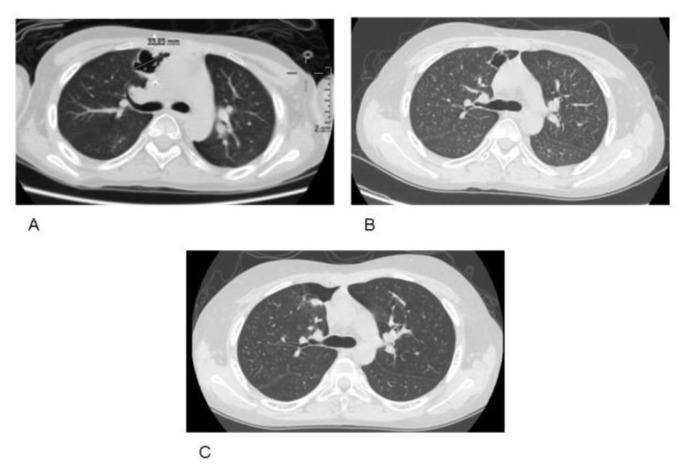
Lung computed tomography CT scan image: (A) large paracardiac rounded cavitary lesion (diameter 3 cm) in the right upper lobe, consistent with invasive pulmonary aspergillosis (IPA). (B): persistence of the cavitary lesion after five months of antifungal therapy (C) Complete disappearance of pulmonary aspergillosis, with a fibrotic lesion in place of the previous cystic lesion.
